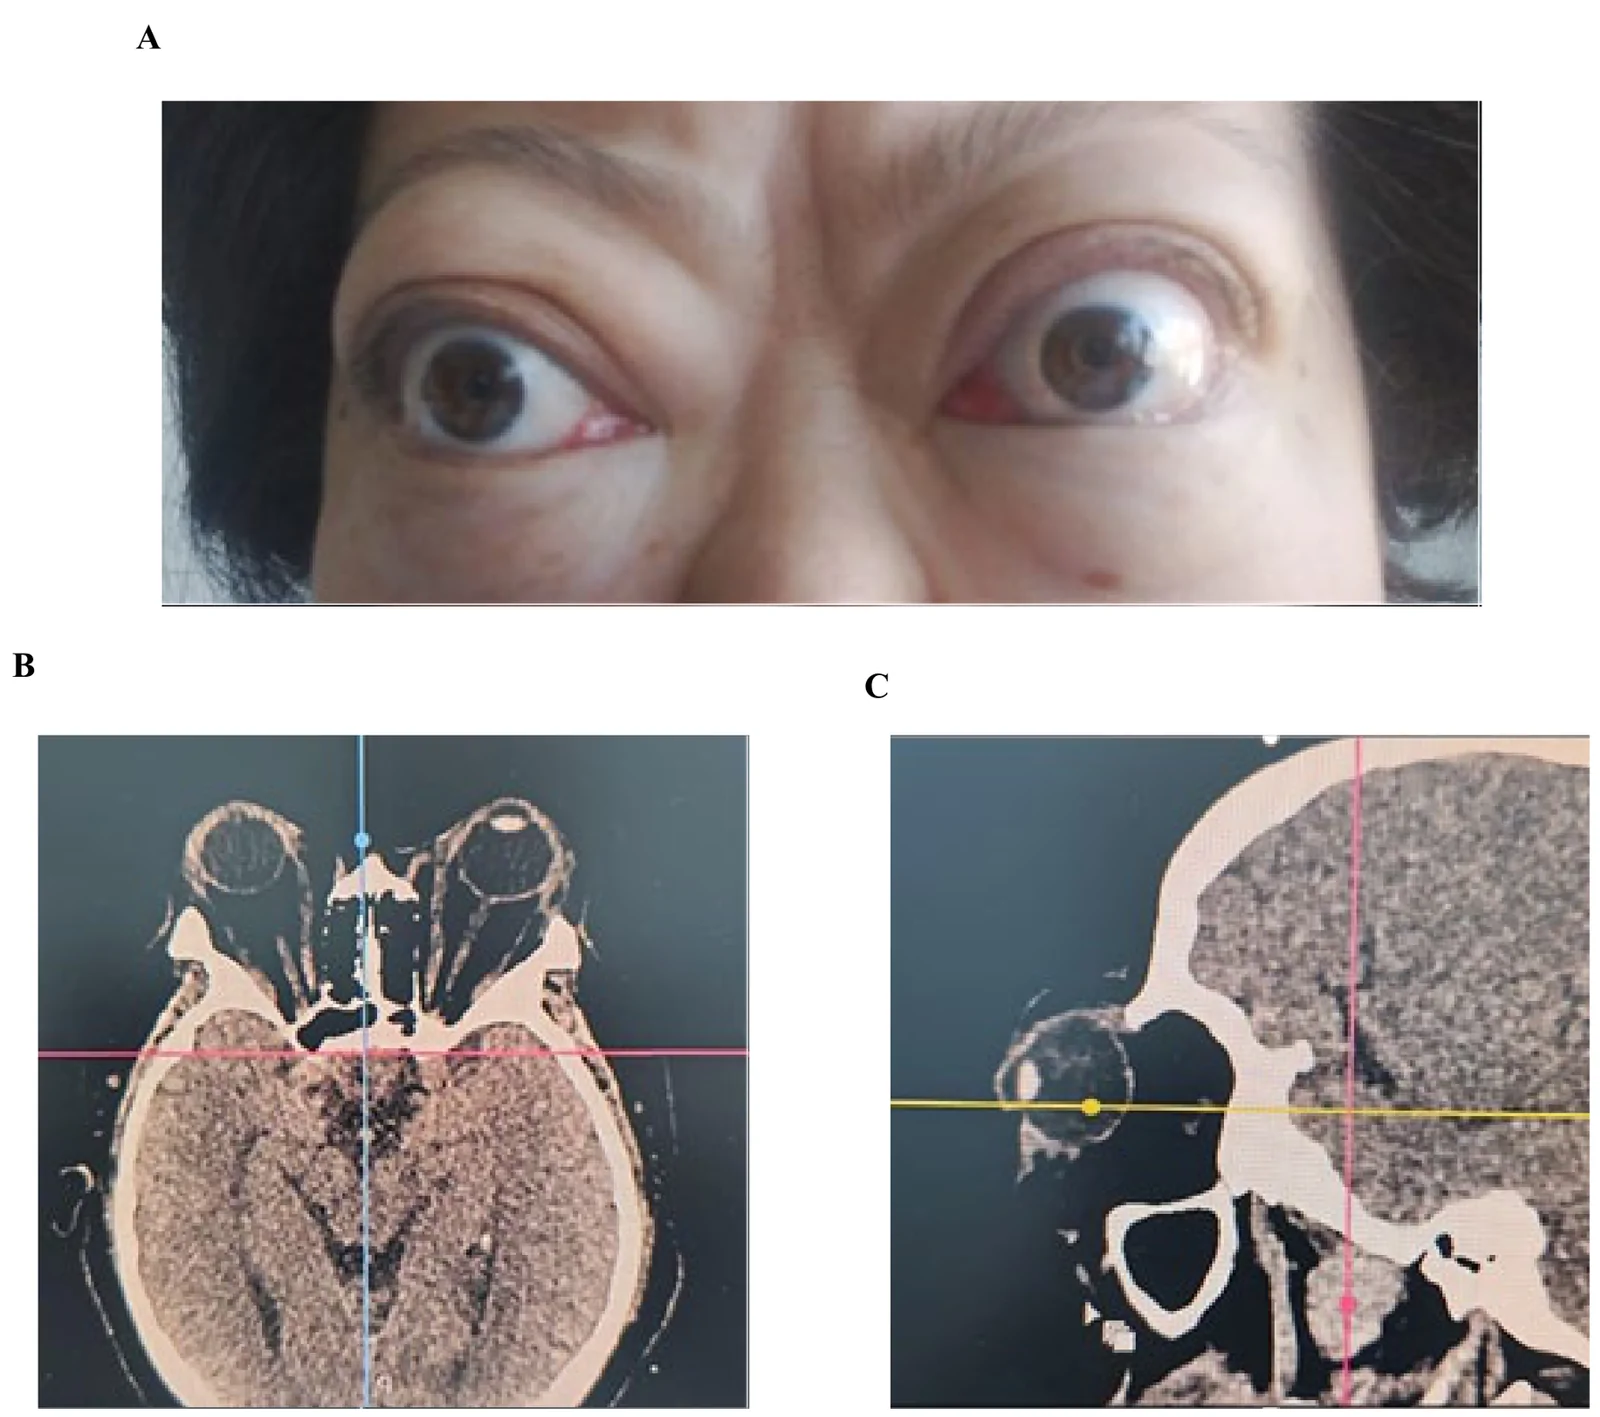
Clinical and imaging presentation of the patient with severe, active, and refractory thyroid eye disease (TED). (A) Clinical photograph of the patient’s ocular region demonstrating advanced bilateral exophthalmos, pronounced upper eyelid retraction, periorbital edema, and conjunctival/caruncular injection. (B) Axial and (C) sagittal computed tomography (CT) scans of the orbits, showing severe bilateral exophthalmos, significant hypertrophy of the extraocular muscles (predominantly the medial recti), marked expansion of retrobulbar orbital fat, and narrowing of the retrobulbar space. These findings collectively highlight active, chronic inflammatory and fibrotic remodeling within the retrobulbar compartment.
